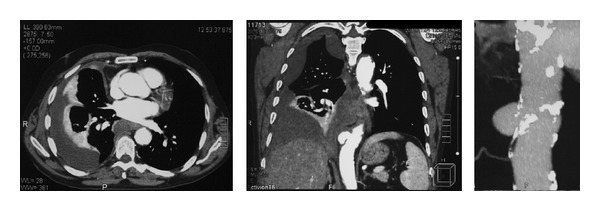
Computed tomographic scan of the chest depicting the right pleural effusion along with atelectasis and the saccular aneurysm of the descending aorta.
